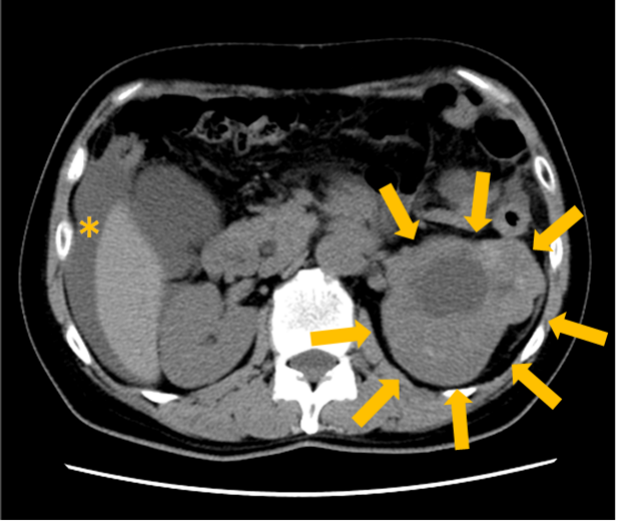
CT image. Arrow: left kidney tumor with internal degeneration. *Ascites.
